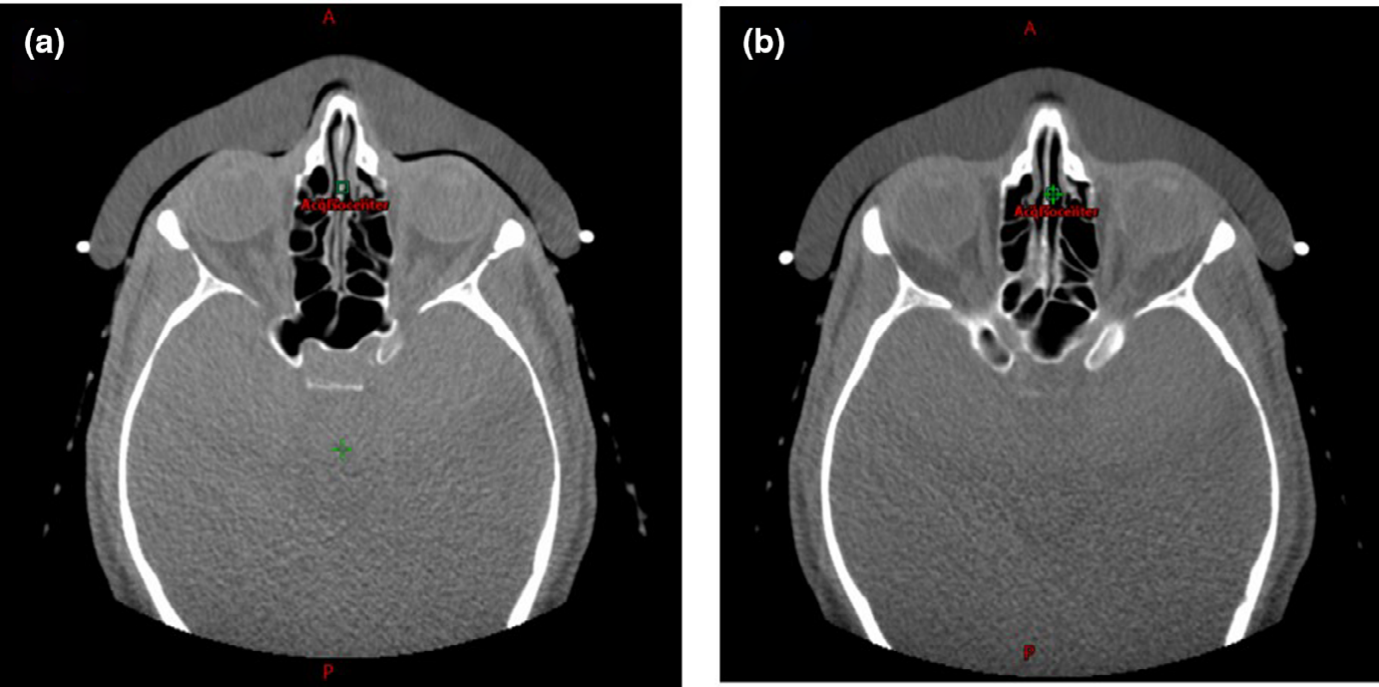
Daily CBCT images of daily setup: (a) a misalignment was identified from an air gap between the bolus and the patient face, (b) the corrected placement of bolus with accuracy of ≤1mm.
